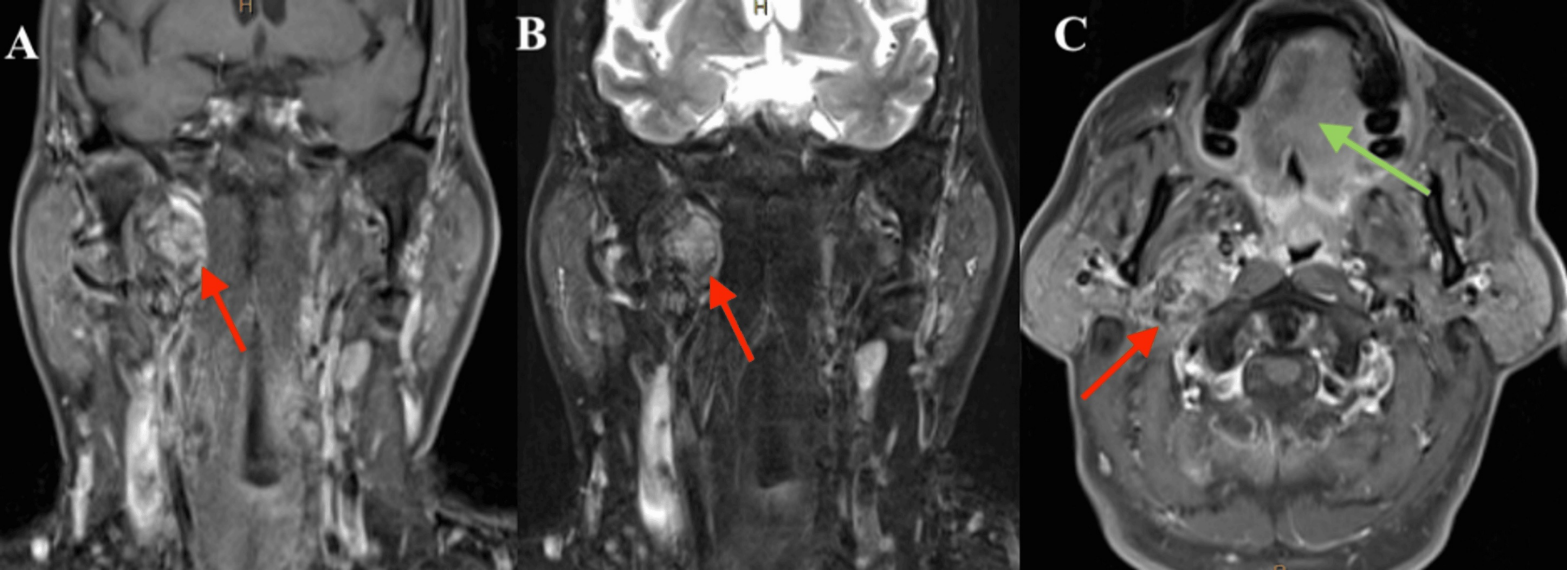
Magnetic resonance imaging depicting a heterogeneously enhancing mass (red arrows) with anterior displacement of the internal carotid artery. The right internal jugular vein flow void is inseparable from the mass. There is notable fatty atrophy and edema involving the intrinsic musculature of the right tongue (green arrow). A) Coronal, T1 sequence post-contrast. B) Coronal, T2 sequence. C) Axial, T1 sequence post-contrast.
